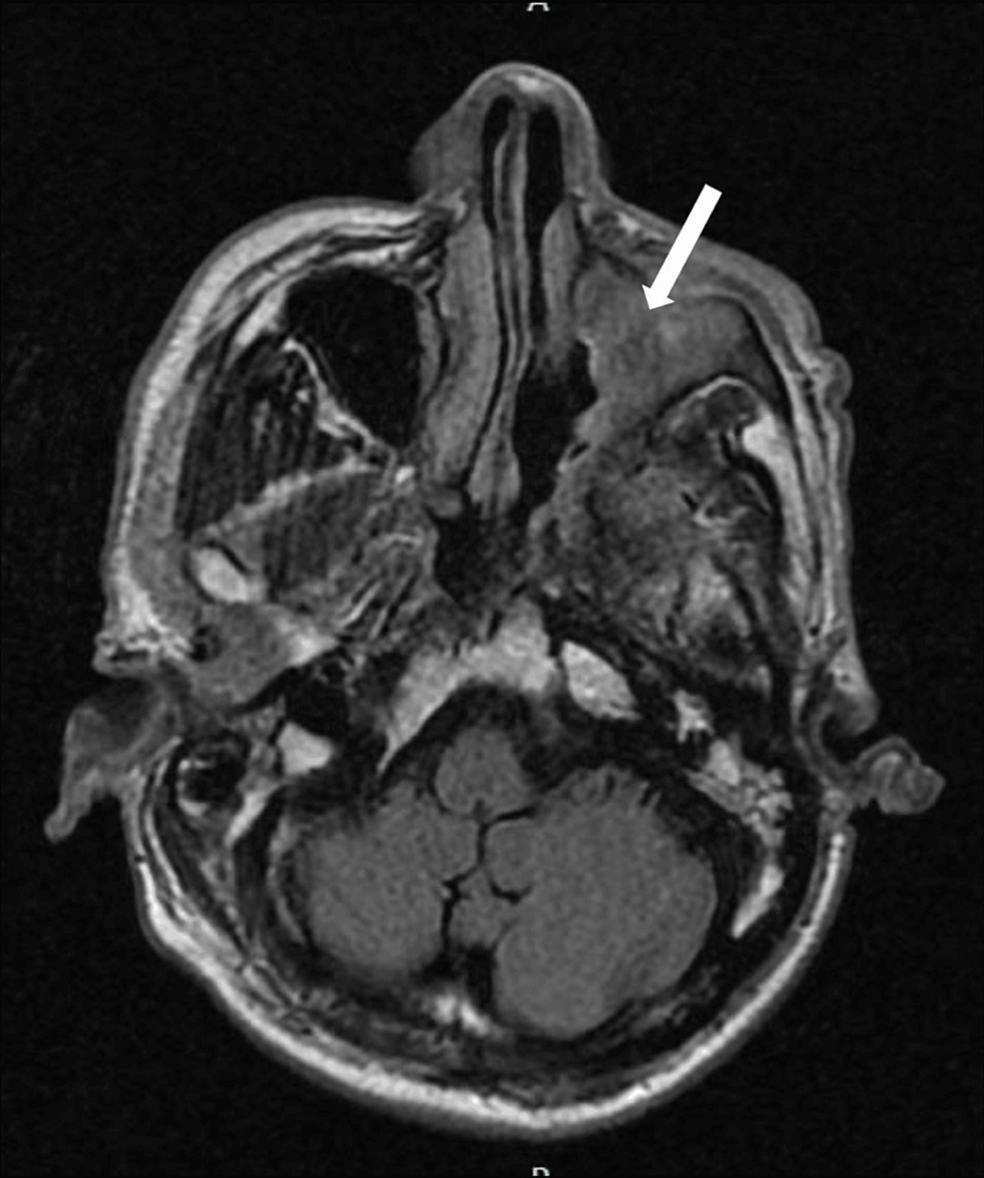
T2-Flair gadolinium-enhanced magnetic resonance imaging (MRI) scan of the head and neck. The white arrow shows the occupation of the left maxillary sinus by heterogeneous material that isointense to the rest of the mucosa.
